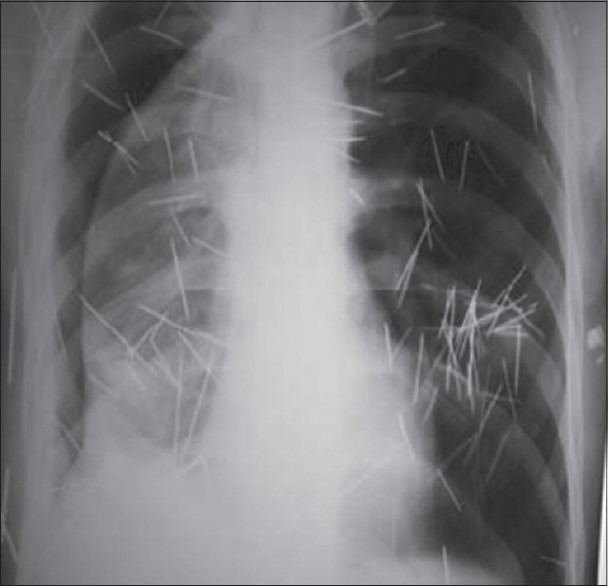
Postoperative chest X-ray after 10 days
